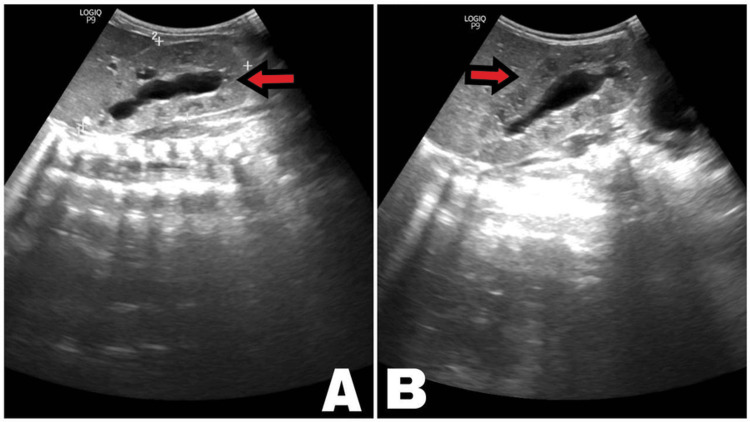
Renal ultrasound A: right kidney, B: left kidney The image shows enlarged kidneys for age (right 7.6 cm x 4.4 cm, left 8.4 cm x 4.1 cm) with a mild increase in cortical echogenicity and hydronephrosis (right 1.7 cm, left 1.8 cm).
